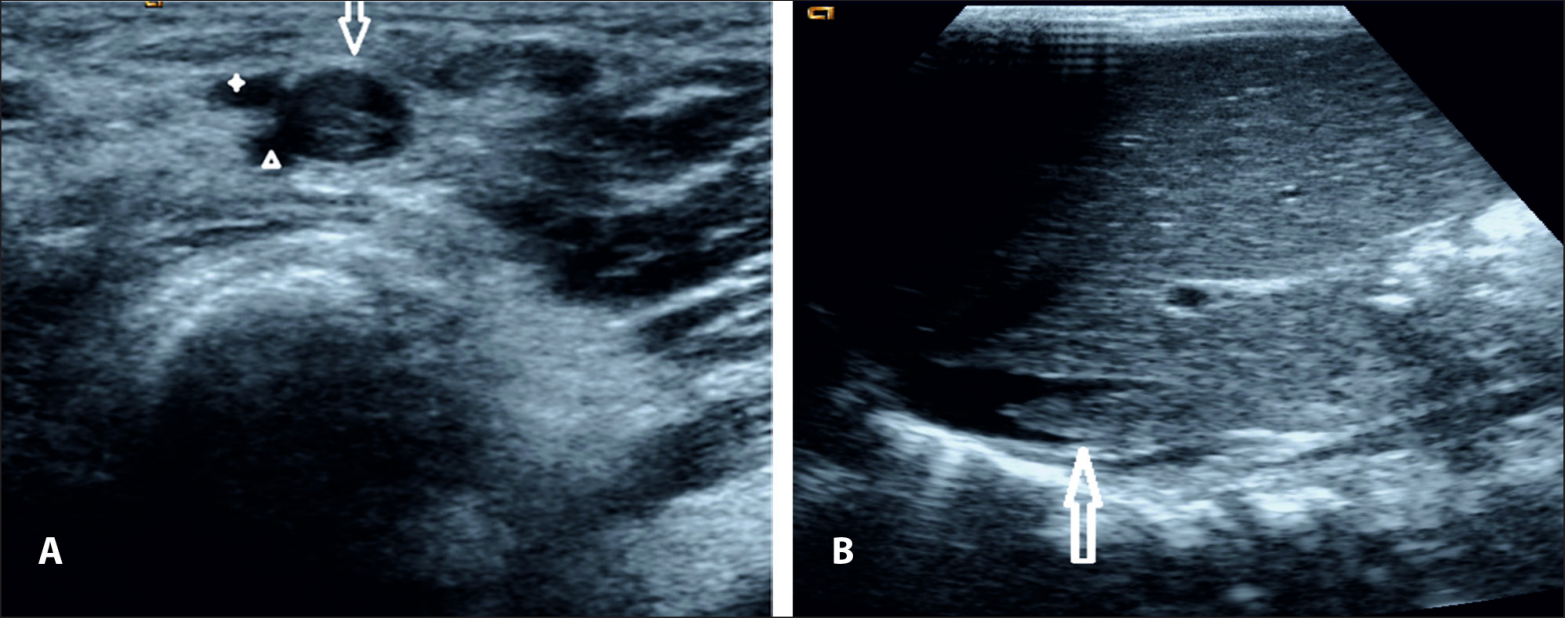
Ultrasound image showing (A) echogenic thrombus (arrow) in the infrarenal IVC and (B) a thrombosed common femoral vein (arrow) adjacent to superficial (asterix) and deep (arrowhead) femoral arteries.
